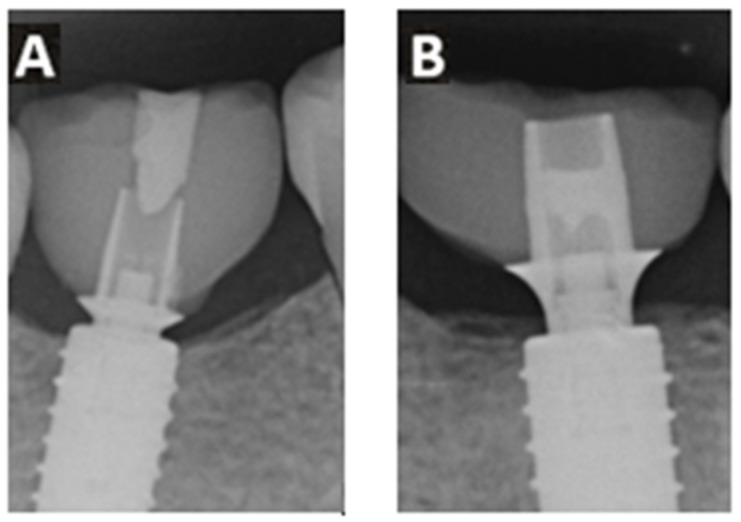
Example X-ray images of the two different Ti-base adhesive bases with gingival height 0.8 mm (A) and 2.0 mm (B)—after three years.
